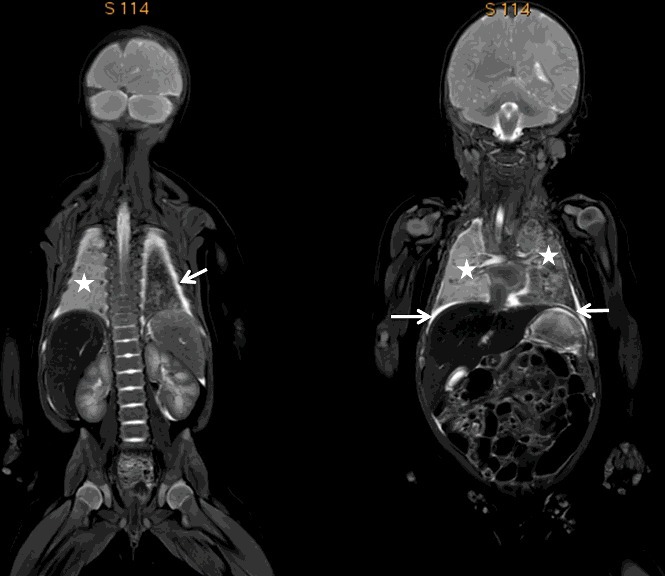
Coronal total body T2-weighted images of a 3-month-old female in the context of SIDS (sudden infant death syndrome) showing slight bilateral pleural effusions (white arrows) and bilateral lung parenchyma consolidations (white stars). The morphology of the abdominal organs is easily identified.
